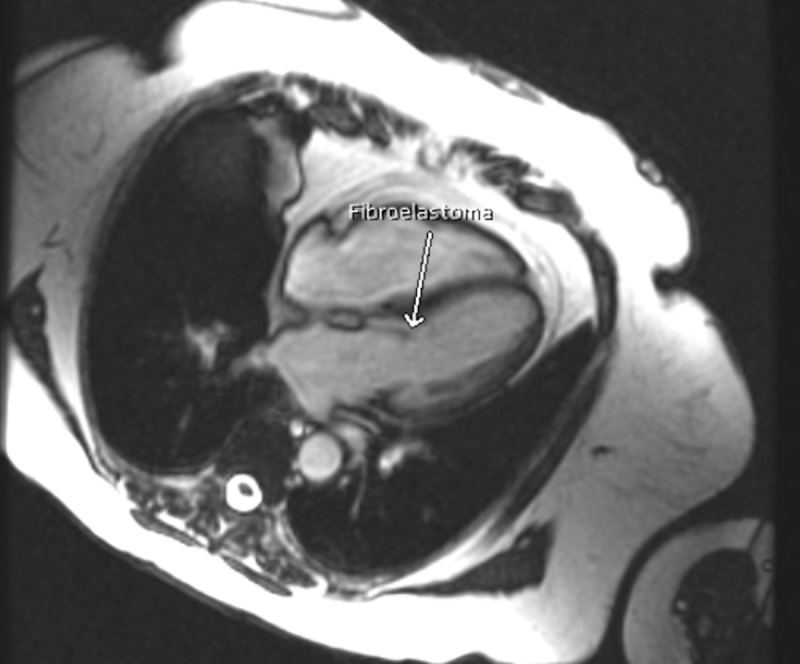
Coronal section of the heart: cardiac MRI (CMR) showing fibroelastoma attached to the subchordal apparatus of the anterior mitral valve leaflet MRI: magnetic resonance imaging; CMR: cardiac magnetic resonance imaging
